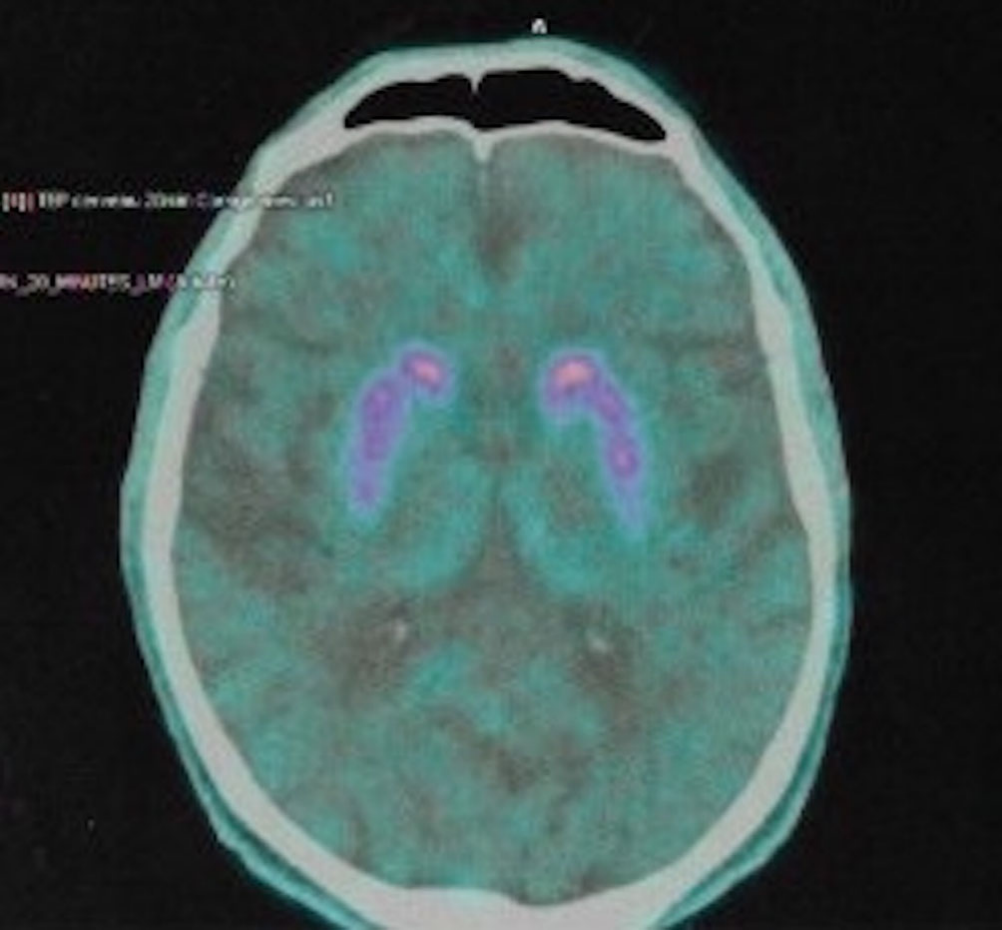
DaT-SCAN SPECT from patient #1, showing reduced striatal uptake, asymmetric, predominant in putamen.
